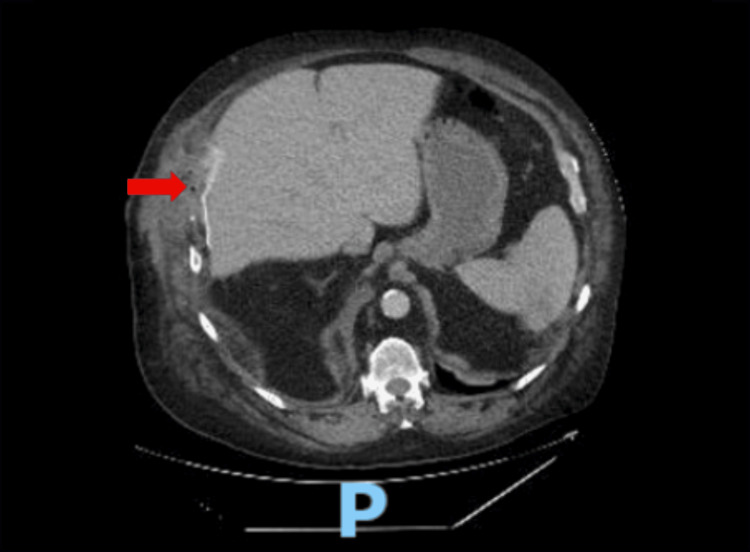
Axial computed tomography image showing surgical repair of the liver herniation Red arrow: Area of diaphragmatic mesh repair.
